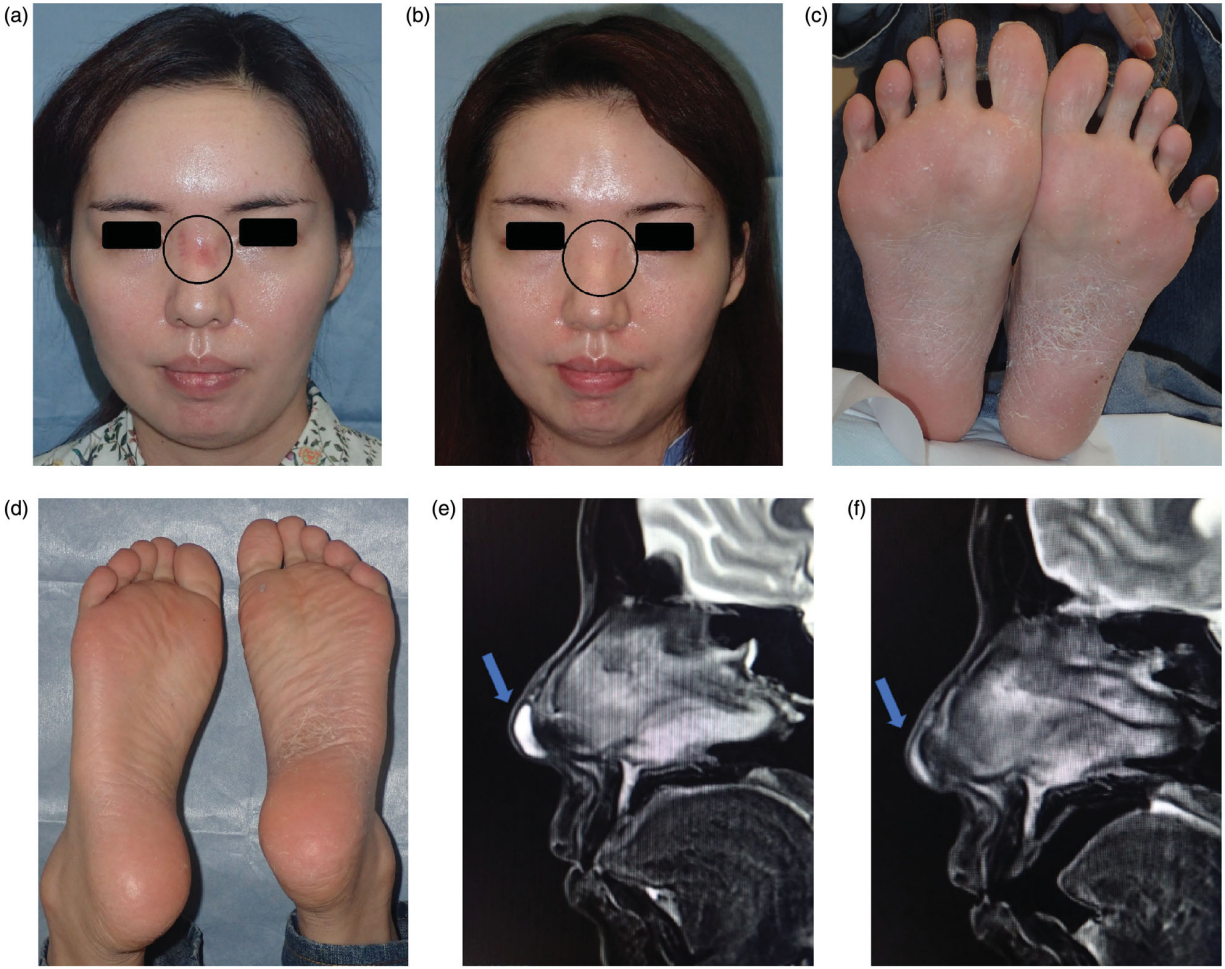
(a) Findings on the first examination: Redness and capillary dilatation were observed in the nose root region over the dorsum of the nose, indicated by the circle. (b) 1 year after surgery: Redness and capillary dilatation in the nose root region disappeared, indicated by the circle. (c) First examination at our hospital: Photograph of the sole. Pustules associated to scaling plaques and some fissures in the palmoplantar area were noted. (d) 1 year after surgery: Photograph of the sole. No recurrence of palmoplantar pustulosis was noted at 1 year after surgery. (e) MRI: A water-soluble implant remained in the region, indicated by the arrow. (f) 1 year after surgery: Postoperative reactions were noted in the region, indicated by the arrow.
